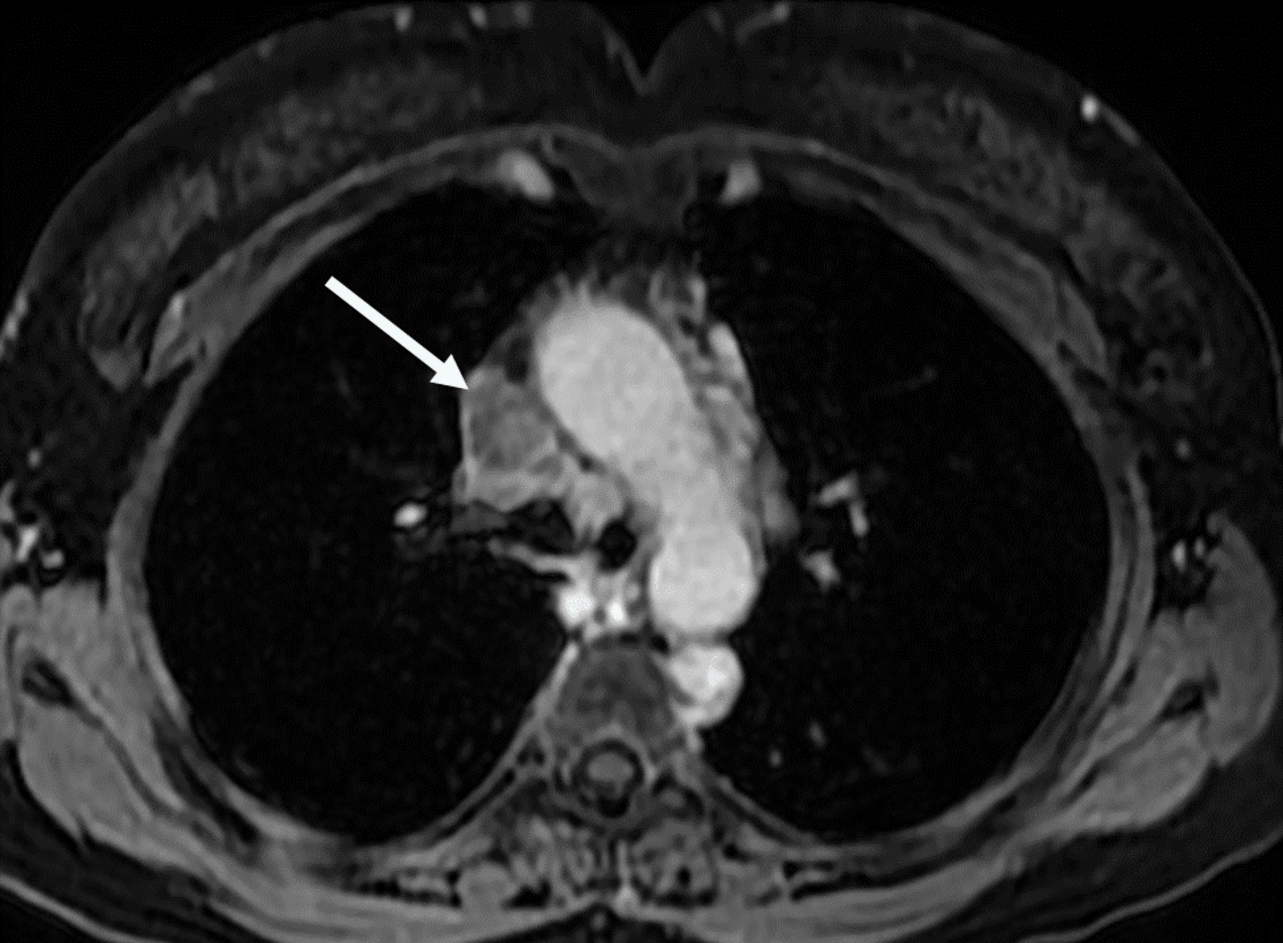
Thoracic MRI in T1 fat-saturated sequence with gadolinium injection: Circumferential parietal thickening of the SVC that moderately enhances with gadolinium injection, suggesting an inflammatory process SVC: superior vena cava
